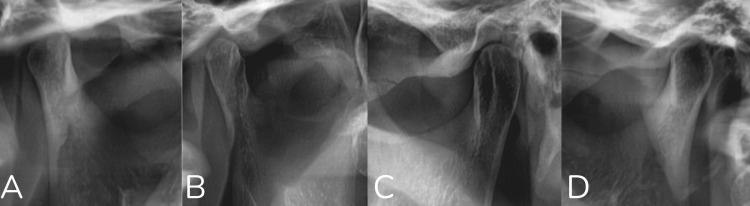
Radiographic classification of mandibular condylar morphology on panoramic radiographs. (A) Flat condyle, characterized by a flattened superior surface.
